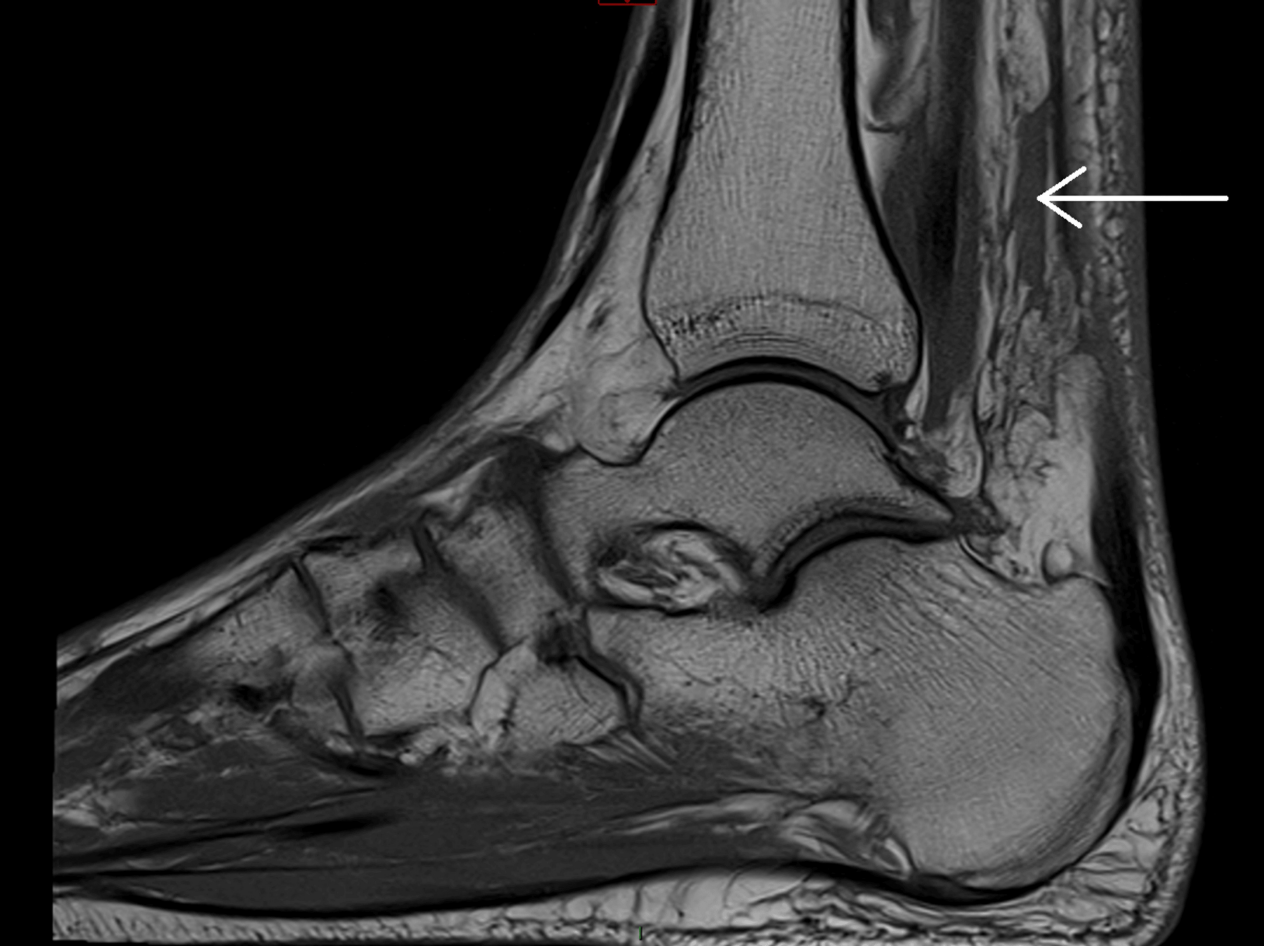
Left ankle MRI – 4 cm gap showing Achilles tendon rupture with local soft tissue inflammatory changes, indicated by white arrow
